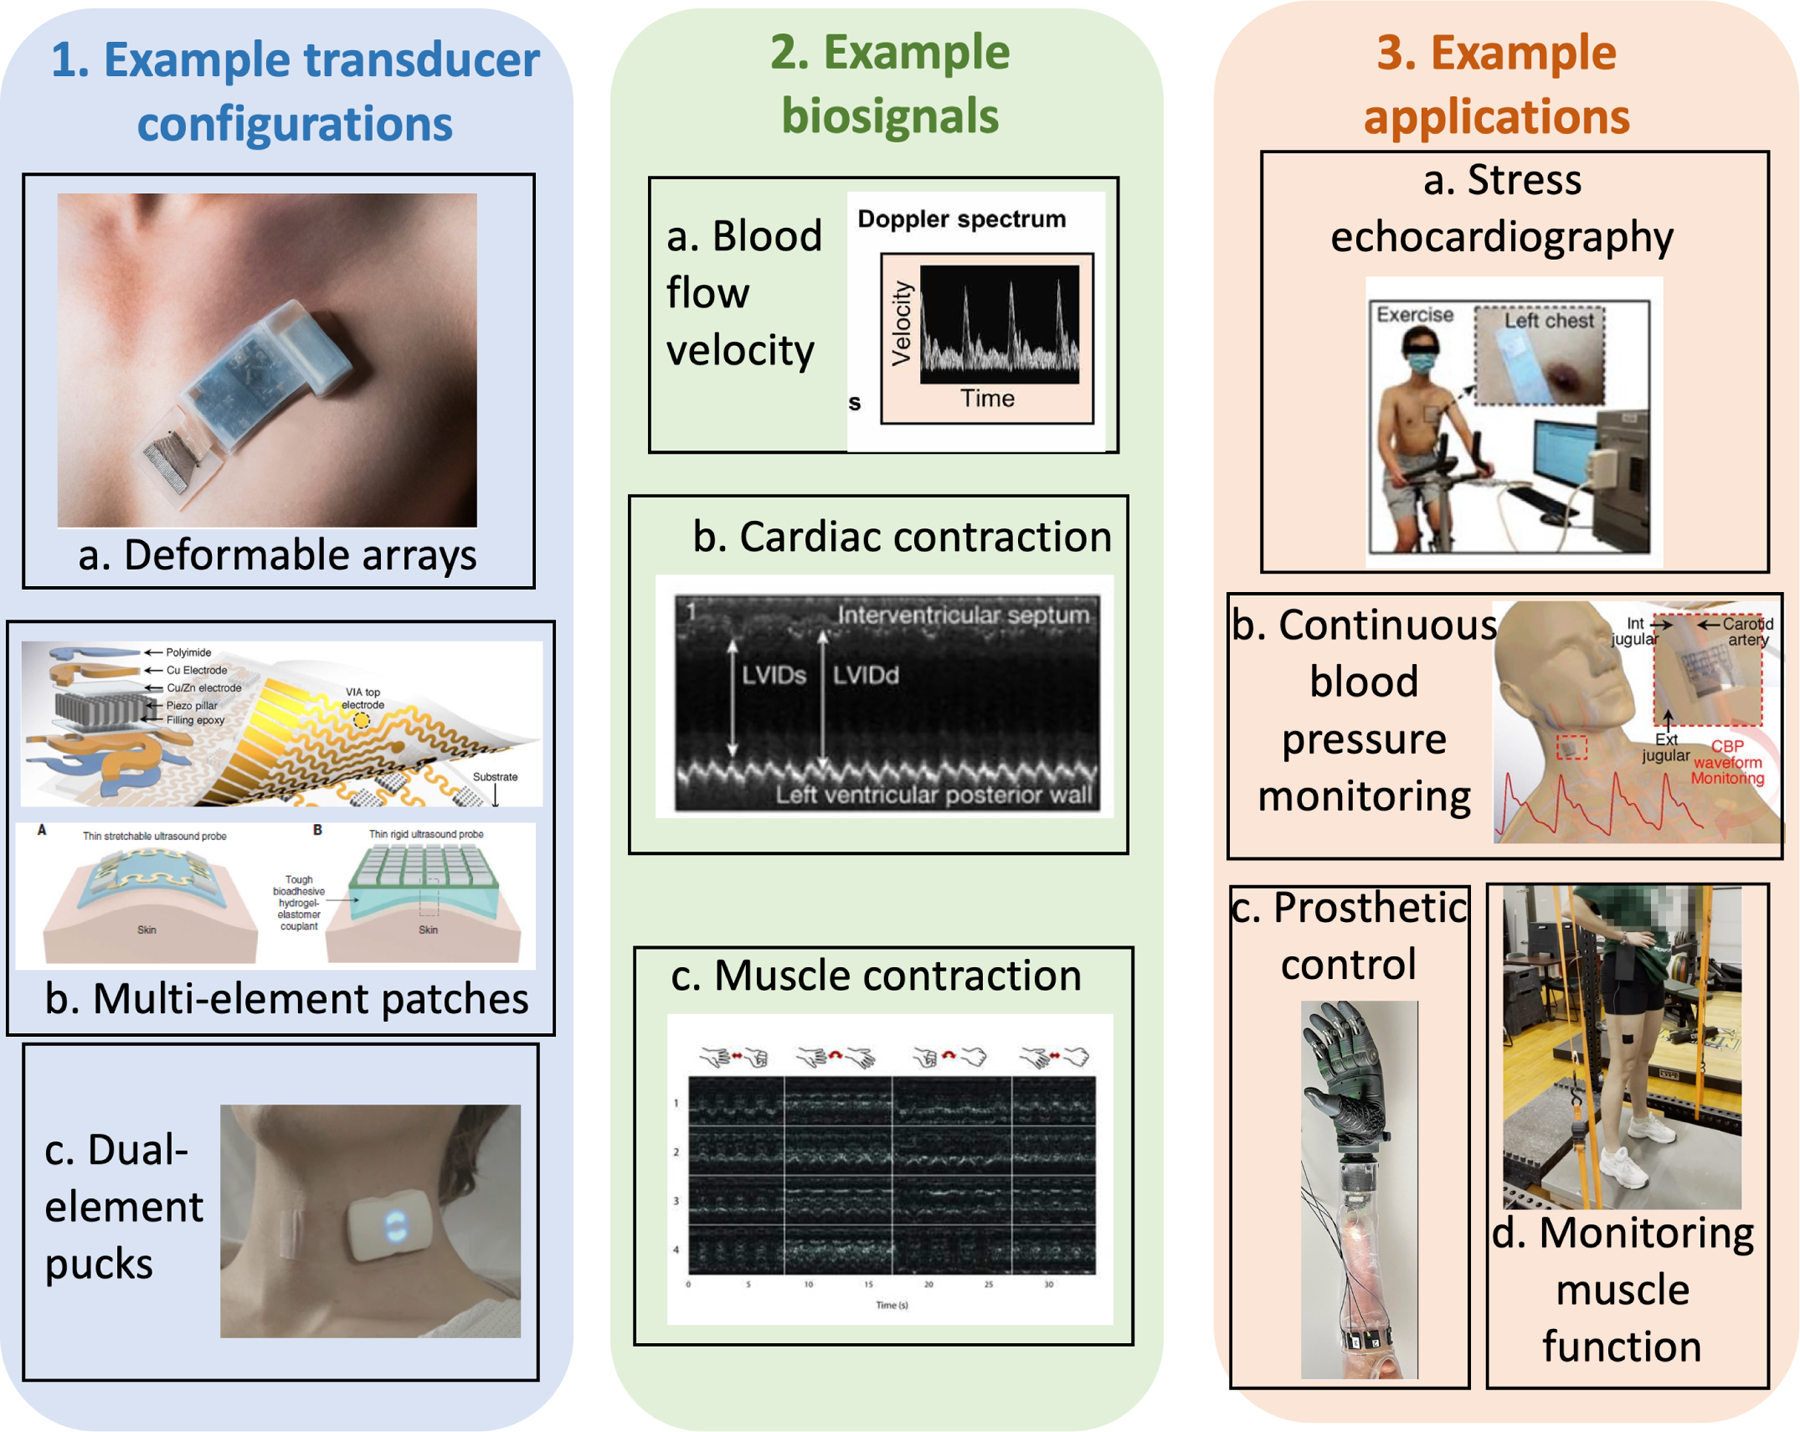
Overview of current state of the art for wearable US. Wearable US systems involve different configurations for transducers, such as deformable arrays [69], multielement patches [8], [18] and single-element pucks [70]; biosignals, such as Doppler spectra [8], cardiac contraction [19] and muscle contraction [53]; as well as applications such as stress echocardiography [8], continuous blood pressure monitoring [20], prosthetic control [60] and muscle function monitoring [70]. Research and development of integrated systems for specific use cases will require interdisciplinary expertise from a range of clinical, scientific and engineering disciplines. All figures were reprinted from their respective journals with permissions. 1. Example transducer configurations—(a) Deformable arrays. (b) Multielement patches. (c) Dual-element pucks. 2. Example biosignals—(a) Blood flow velocity. (b) Cardiac contraction. (c) Muscle contraction. 3. Example applications—(a) Stress echocardiography. (b) Continuous blood pressure monitoring. (c) Prosthetic control. (d) Monitoring muscle function.
